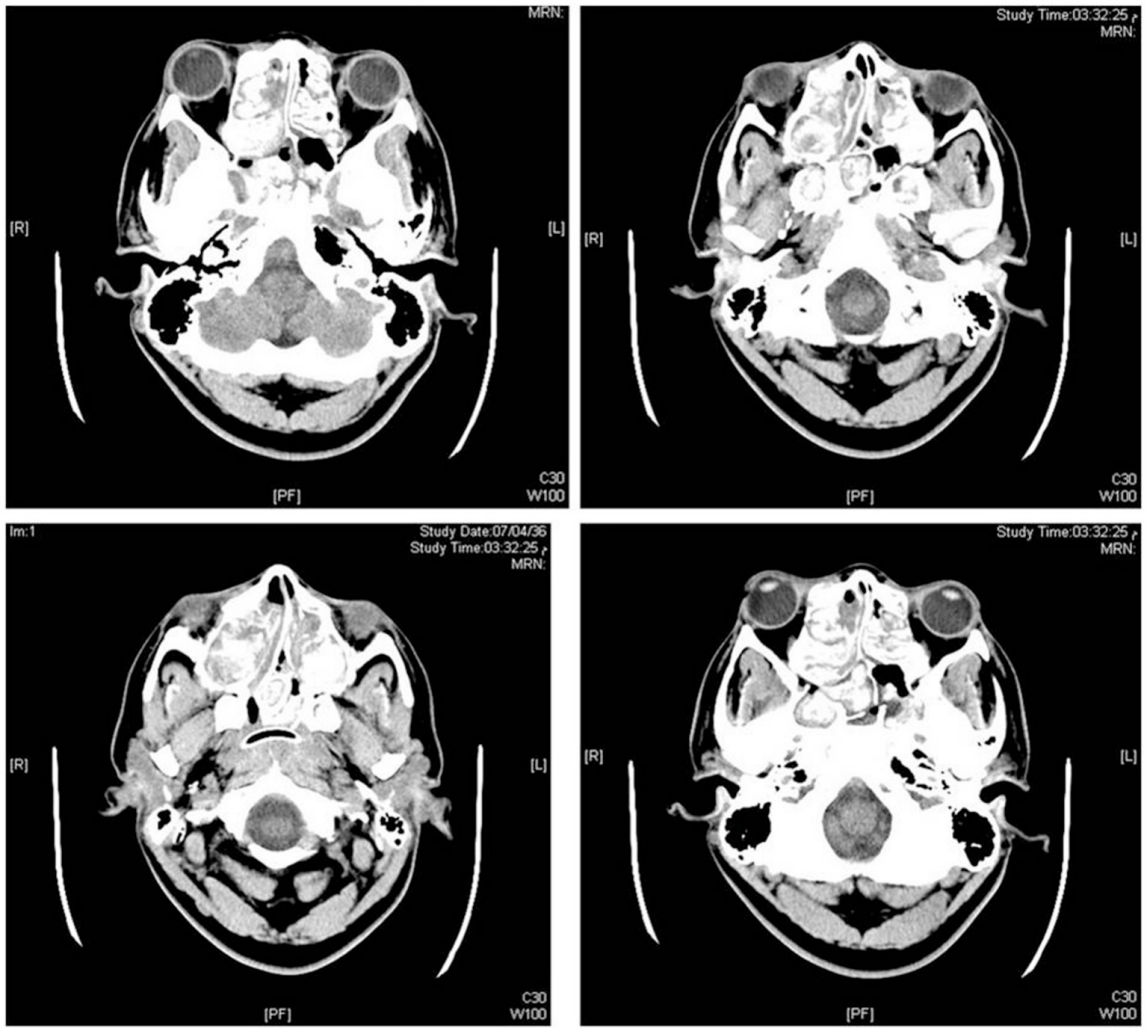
Imaging studies. Computed tomographic (CT) images of the head without administration of contrast, showing ethmoidal, sphenoid, and maxillary sinuses occupied by hyperdense soft tissue suggesting fungal sinusitis (bilateral sinupolyposis).
